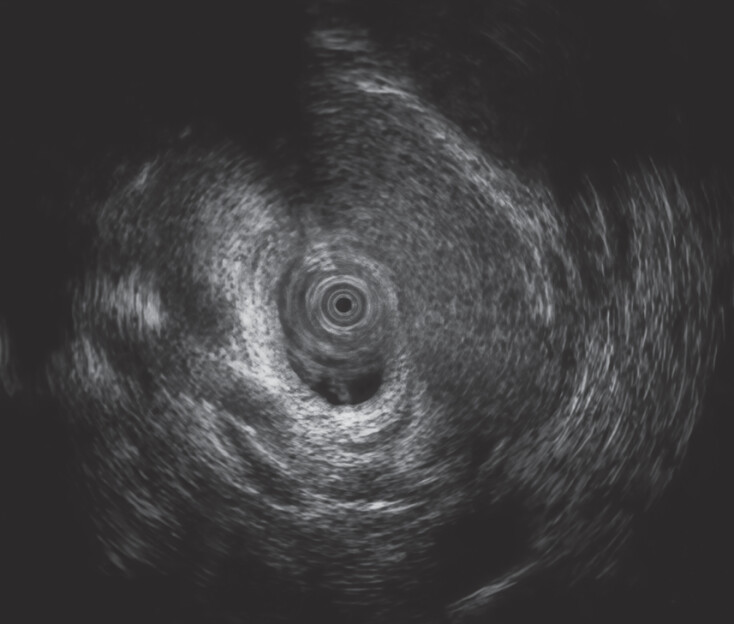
Endoscopic ultrasonography revealed bile duct wall
